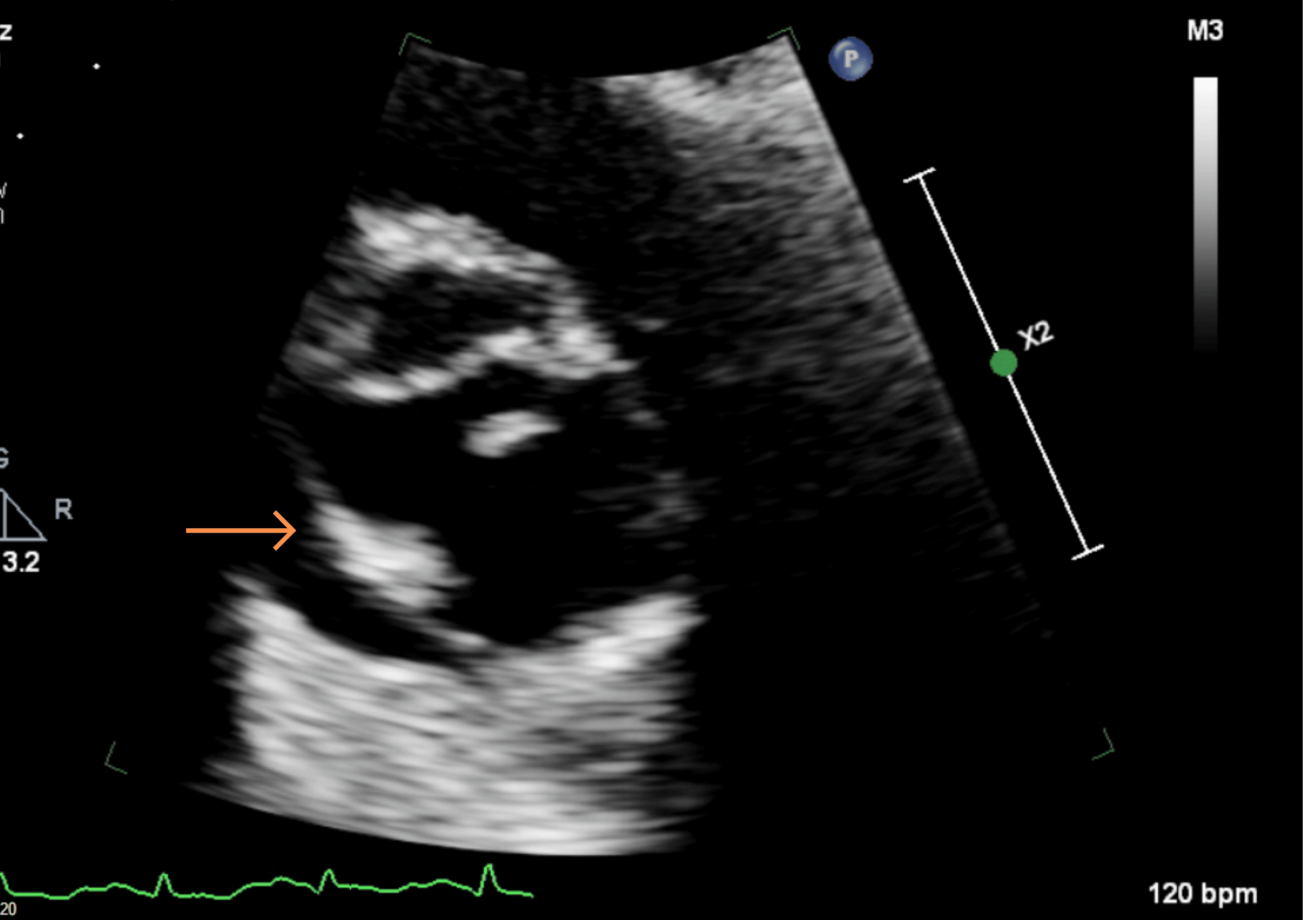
TTE PSAX view - aortic valve and the possible vegetation structure (orange arrow) TTE: Transthoracic echocardiogram, PSAX: Parasternal short axis
